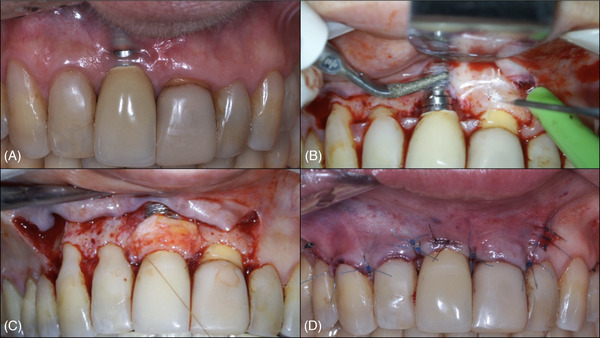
Case 2: Steps of the first surgical intervention. (A) Baseline aspect of the surgical site. (B) Implantoplasty with an ultrasonic diamond tip of higher‐grain size, after incision and flap elevation. (C) Stabilization of the CTG to the interproximal papillae. (D) Coronal advancement of the flap and stabilization with sutures 6.0 blue nylon and 7.0 black nylon at the fenestration.
